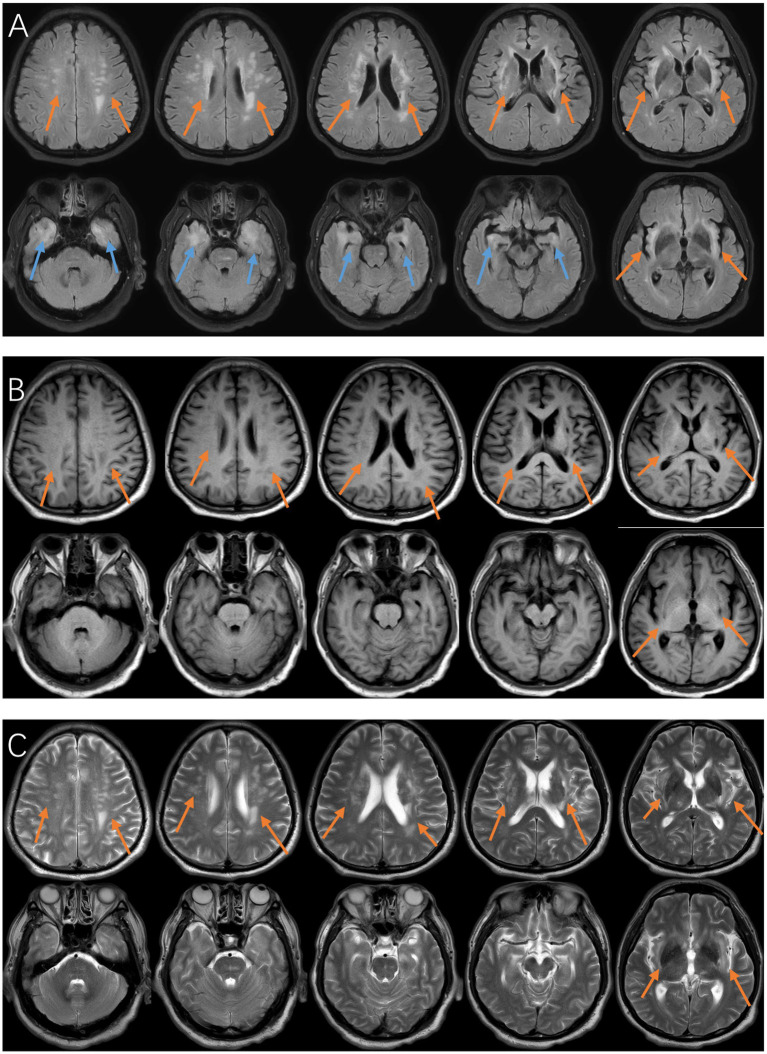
(A) FLAIR, orange arrows show patchy periventricular and centrum semiovale hyperintensities (demyelinating changes); blue arrows show bilateral medial temporal lobe hyperintensities (limbic encephalitis changes). (B) T1, arrows show patchy hypointensities in the periventricular and centrum semiovale regions, indicating underlying tissue injury associated with demyelination. (C) T2, arrows show patchy hyperintensities in the periventricular and centrum semiovale regions, further delineating lesion extent and reflecting increased water content. The combined use of FLAIR, T1, and T2 sequences provides complementary information regarding lesion distribution, extent, and severity.
